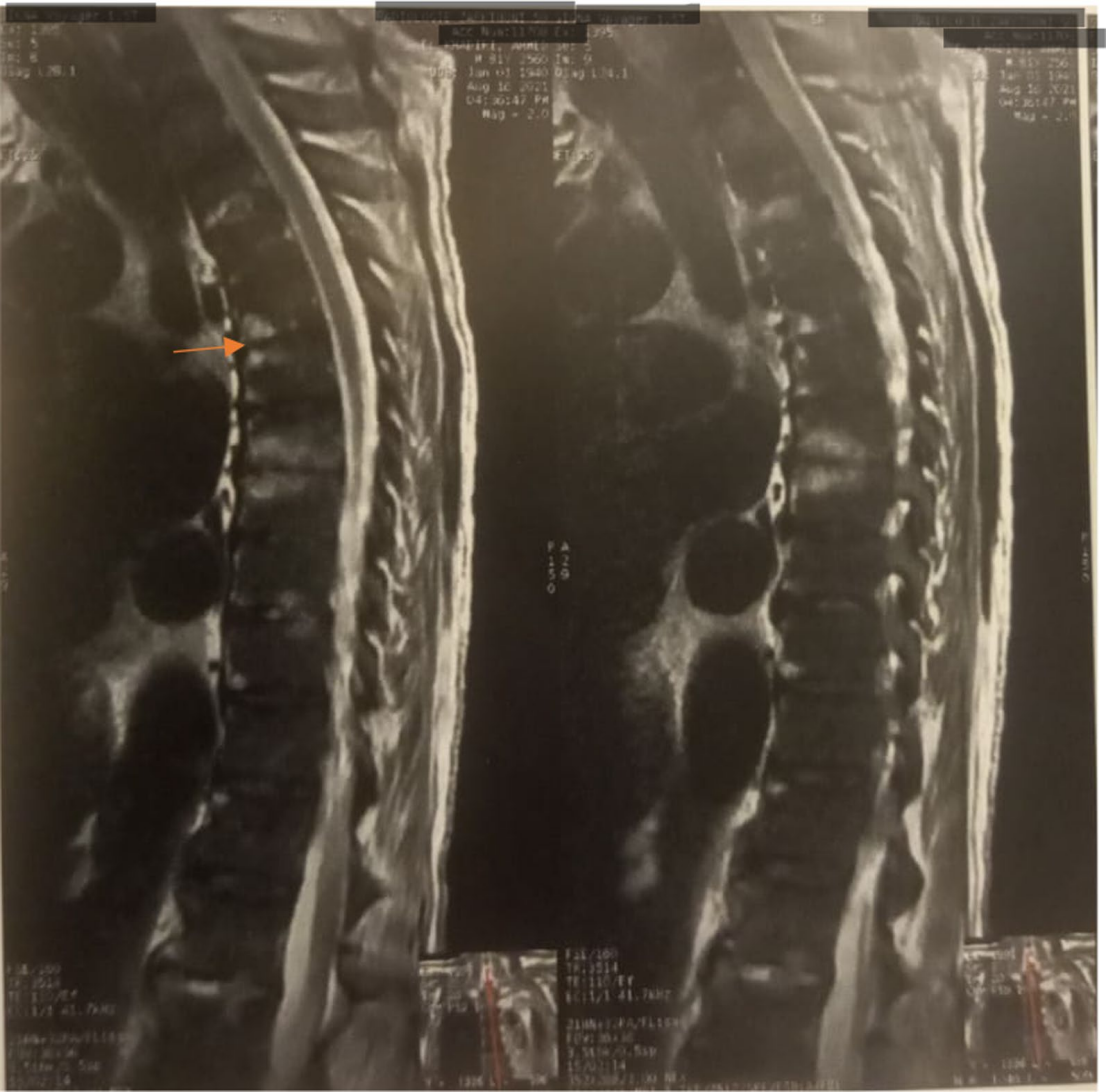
Cervico-dorso-lumbar MRI showing vertebral osteomyelitis
